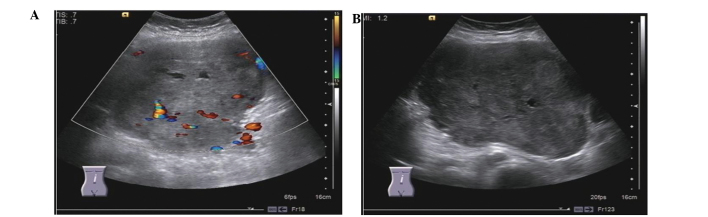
(A) Color Doppler flow imaging revealed some funicular blood flow signals. (B) Abdominal ultrasound showed a large mass with uneven echo and clear edge from the surrounding tissues.
